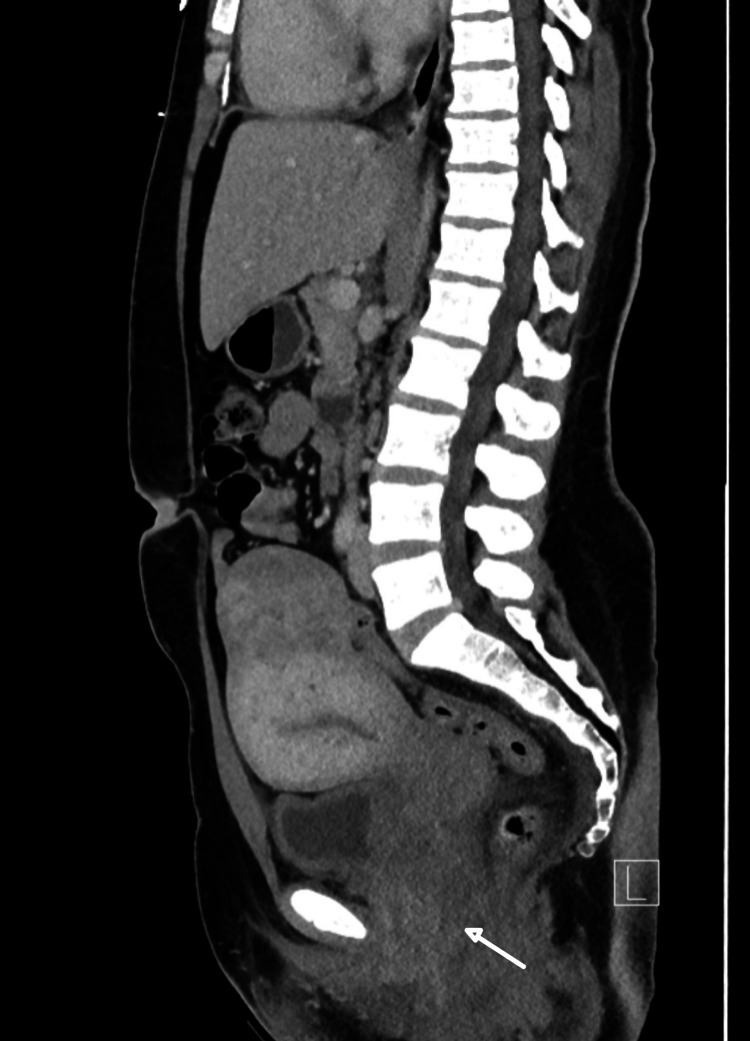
Contrast-enhanced computed tomography of the abdomen and pelvis showing vulvovaginal and cervical thickness with diffuse edema (white arrow) and findings concerning for pelvic inflammatory disease and developing an abscess
